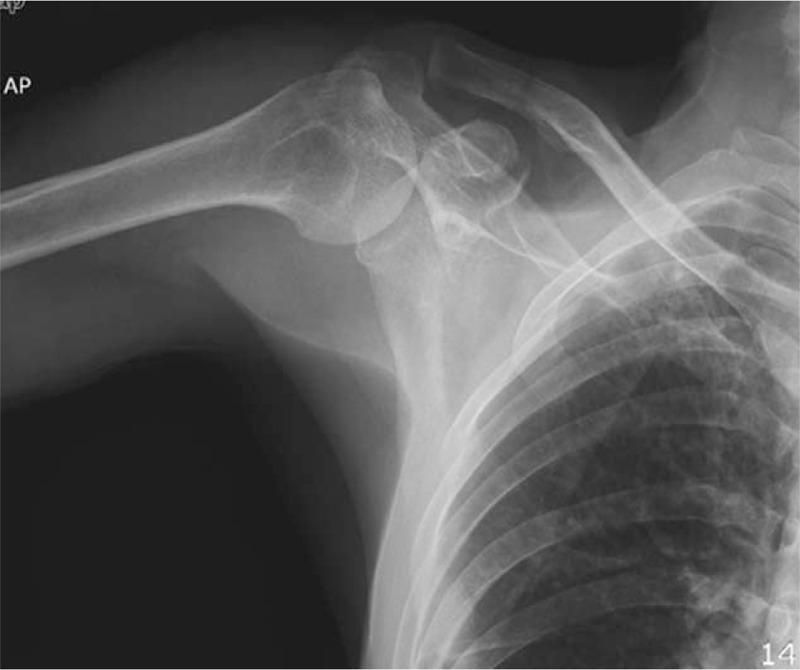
Preoperative anteroposterior X-ray showed CP fracture and a third-degree AC joint separation.
